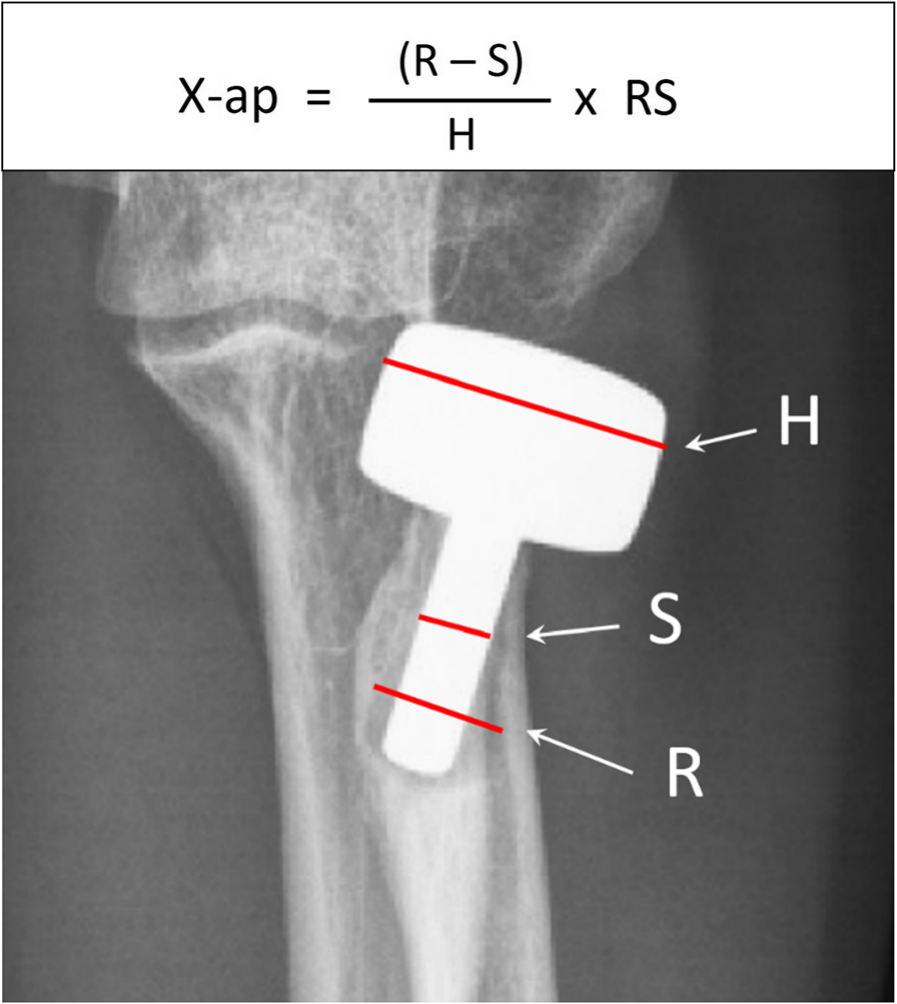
Measurement of periprosthetic radiolucency on anteroposterior view (X-ap) by 3 red lines on radiographs. H red line: width of radial head component on radiographs. S red line: width of stem on radiographs. R red line: maximal width of radiolucency on radiographs. RS: real size in head component of the prosthesis
